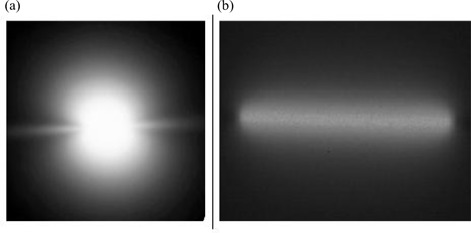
Auto radiographs of (a) a conventional seed‐type ${}^{103}Pd$ source and (b) a RadioCoil ${}^{103}Pd$ linear source (RadioMed Corporation, Tyngsboro, MA) 5.0‐cm long.
